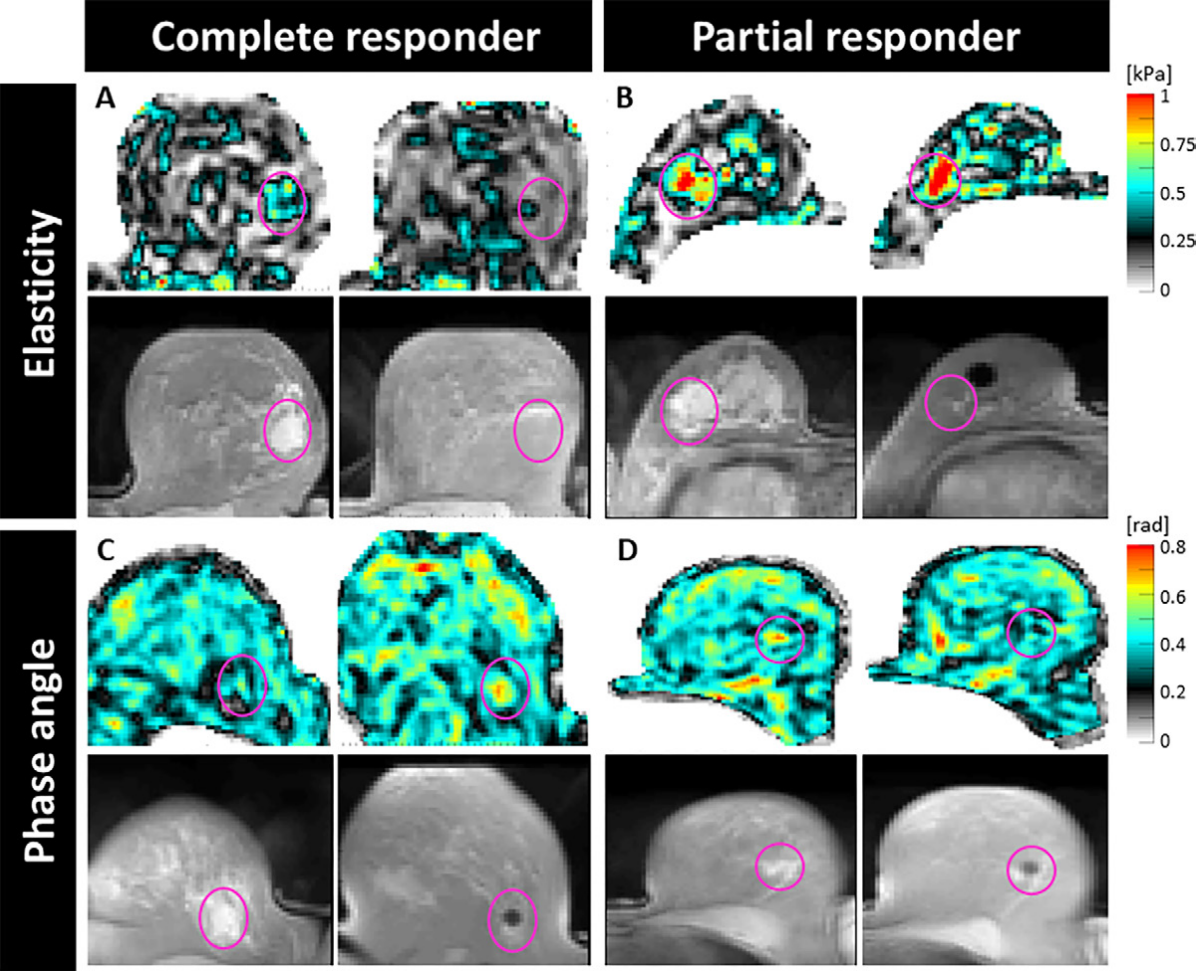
Axial elastograms with corresponding T2-weighted images as examples for
